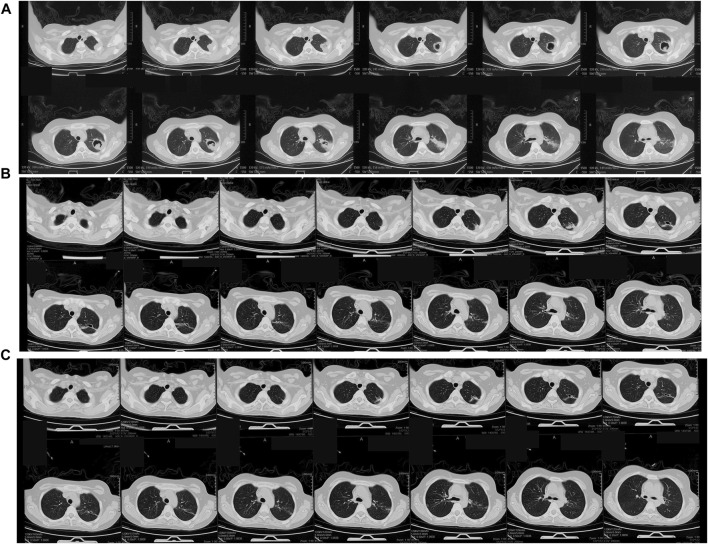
(A) Before treatment of bronchoscopic instillation, chest CT shows a solid left upper lobe shadow with cavity formation visible within it. (B) 55 days after treatment, chest CT shows that the solid shadow in the upper lobe of the left lung is reduced, and the cavity is further reduced. (C) 88 days after treatment, chest CT shows further reduction of solid shadow in the upper lobe of the left lung. Comparison of efficacy between Aspergillus and Mycor patients.
